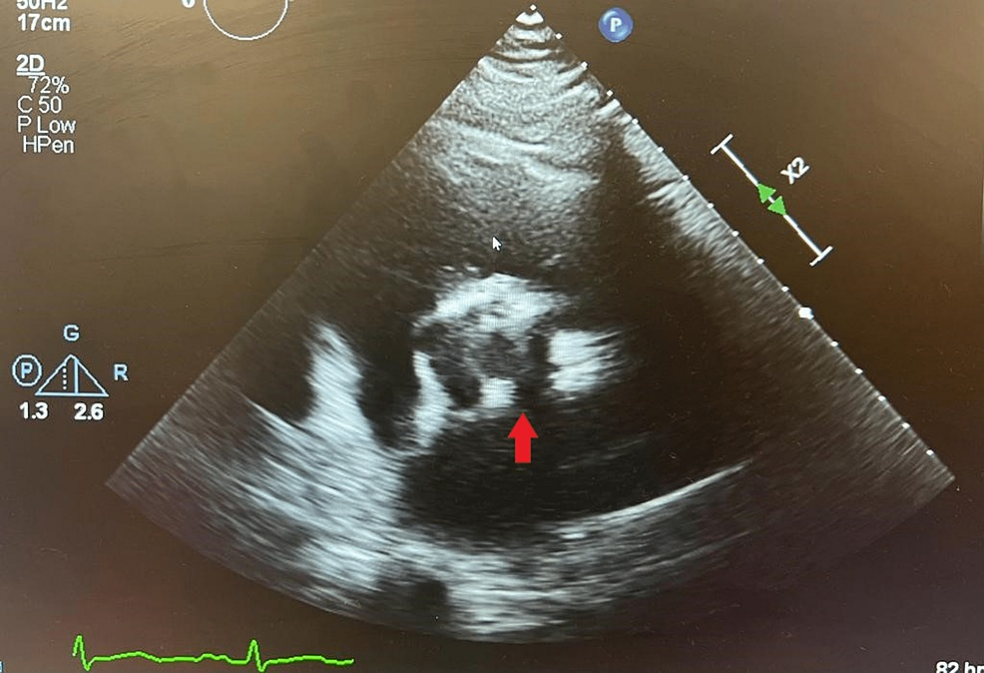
Transthoracic echocardiogram Arrow indicates heavily calcified bicuspid aortic valve
